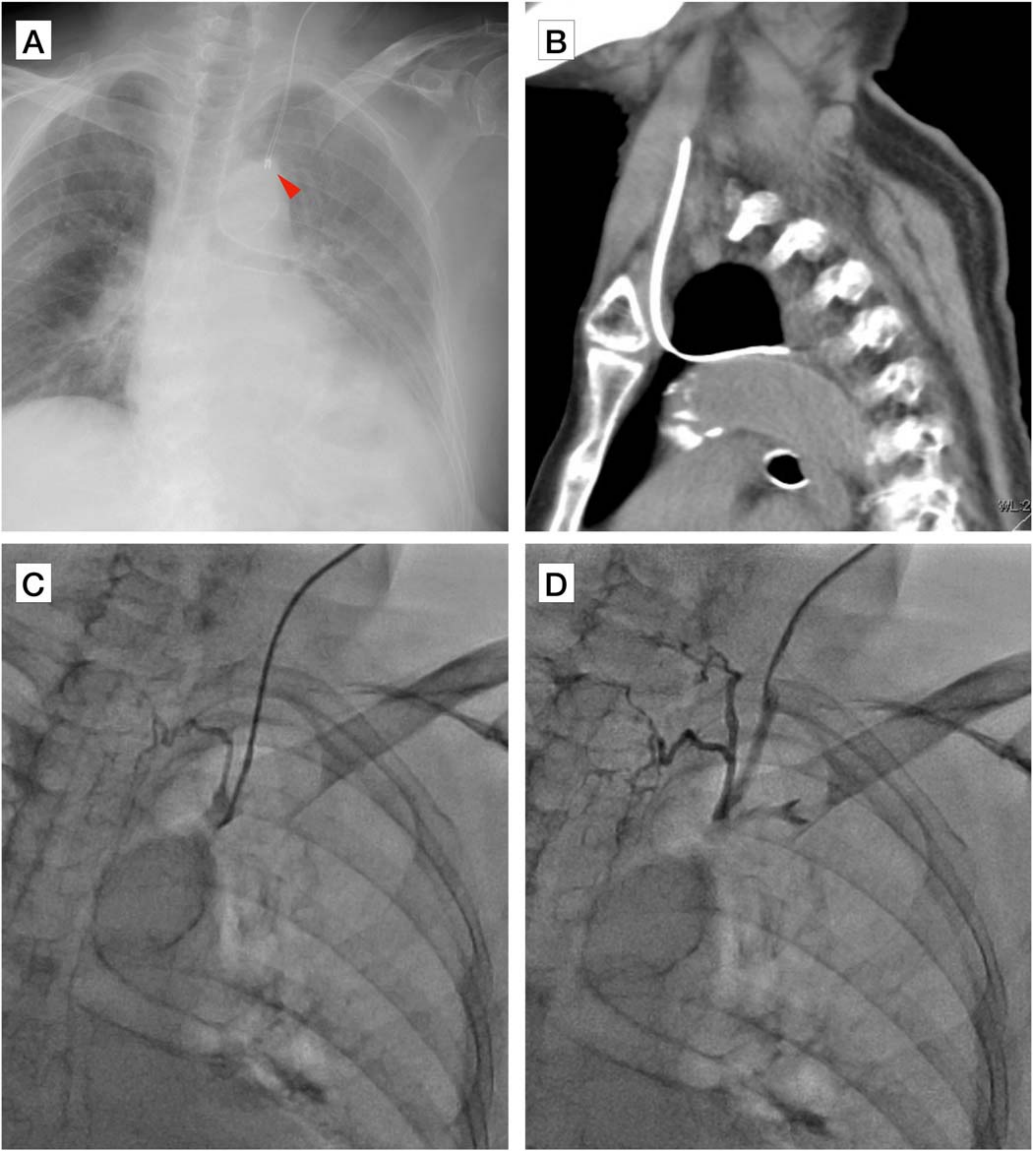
(A) Chest radiograph displaying dotted and displaced central venous catheter with hyperintense images (arrowhead). (B) Computed tomography scan showing the central venous catheter appearing to penetrate the pulmonary apex. (C) Venography through the central venous catheter showing catheter tip in the left superior intercostal vein. (D) Venography through the central venous catheter showing absence of the left brachiocephalic vein with catheter repositioned in the left internal jugular vein.
